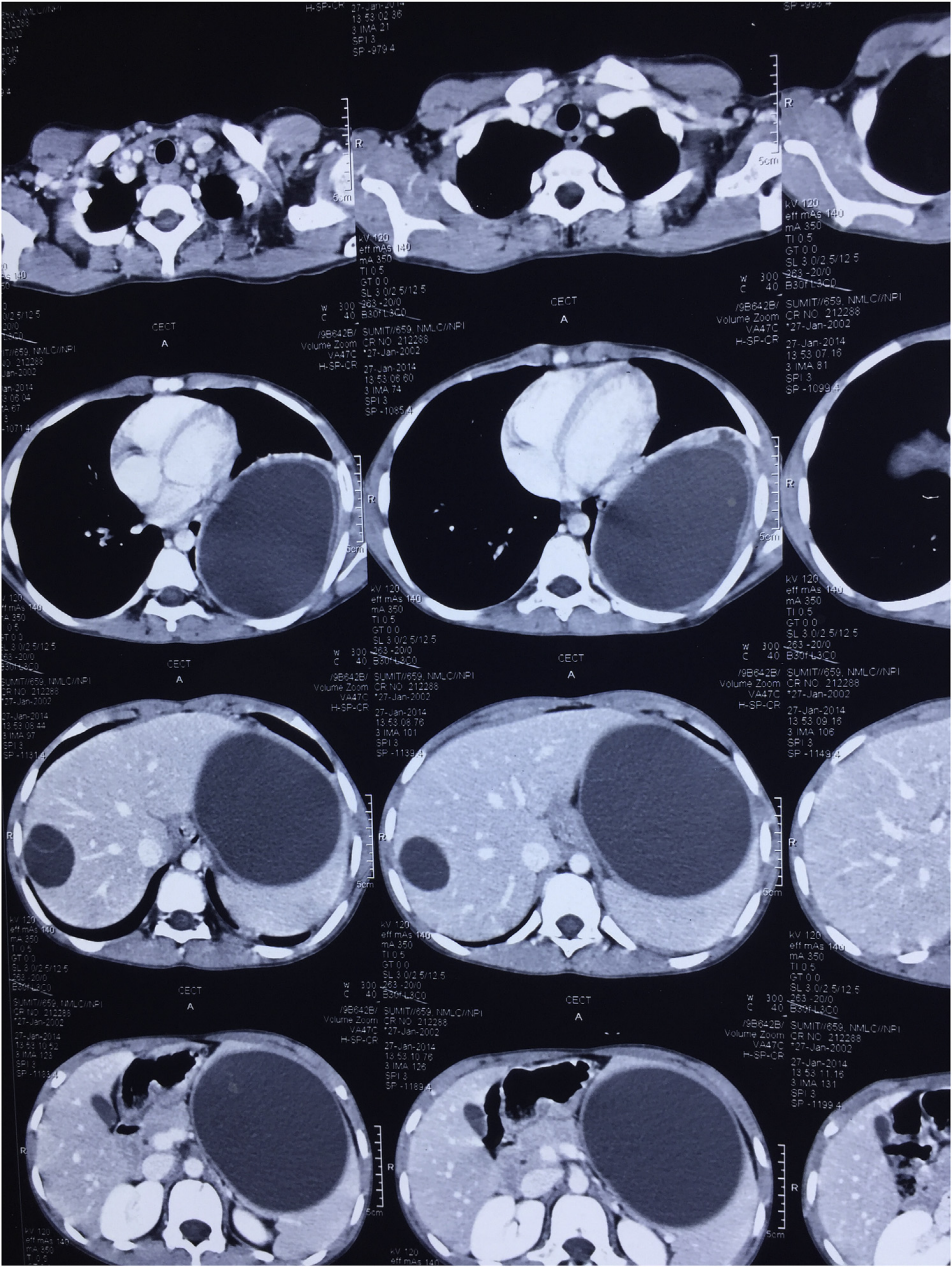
CT image after contrast enhancement showing hydatid cysts in liver, lung and spleen in different sections.
